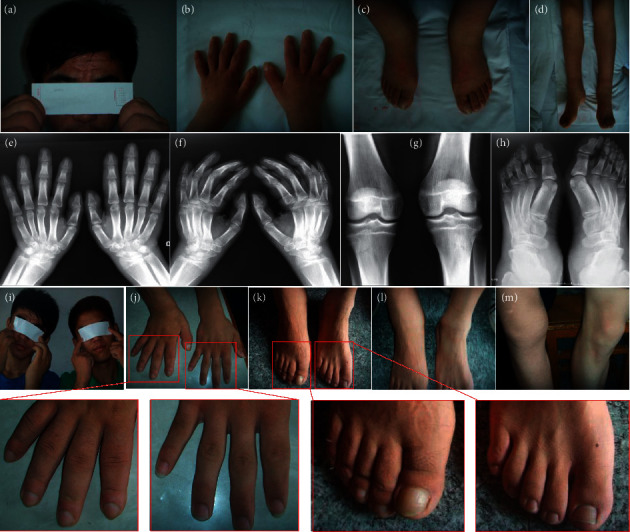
Clinical manifestations of the twin brothers. a–h. Clinical features and radiographs of the proband. i–m. Clinical features of the proband and his twin brother (left, the proband; right, his twin brother). a, i. Thickening and furrowing of the facial skin. b c, j, and k. Digital clubbing of the fingers and toes. d, l, and m. Swelling of the knees and ankles. e–h. Radiographs of the hands, knees, and feet.
